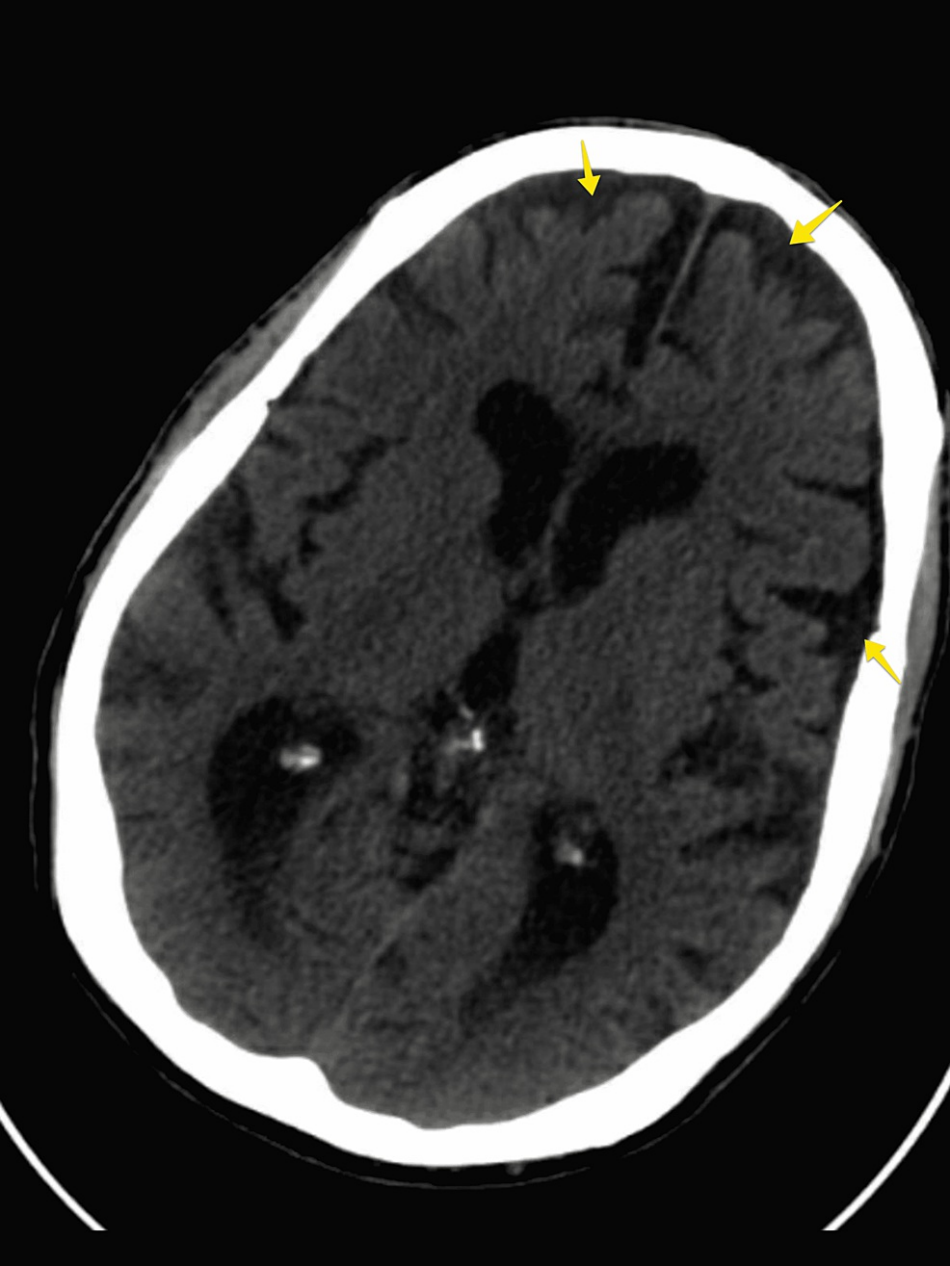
A non-contrast CT scan with arrows highlighting evident cerebral atrophy of the frontal and temporal regions. CT: computed tomography
